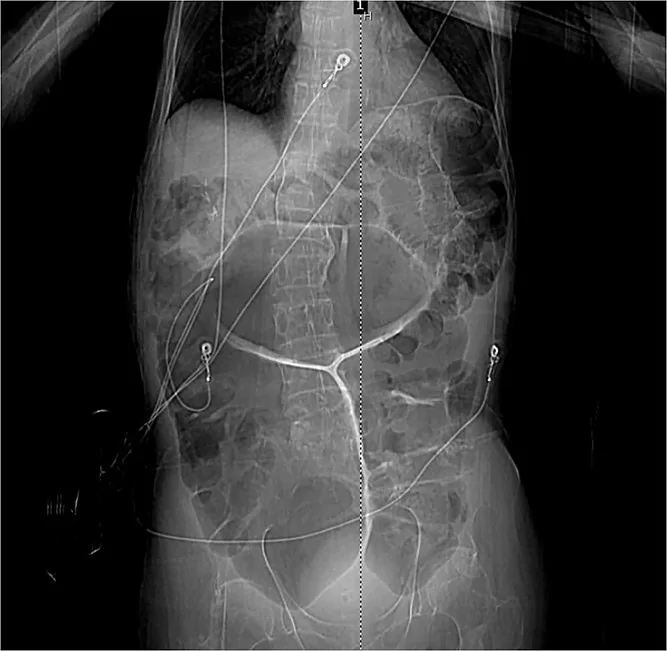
CT scout topogram of the abdomen and pelvis demonstrating marked colonic dilatation with haustral markings, consistent with large bowel obstruction.
